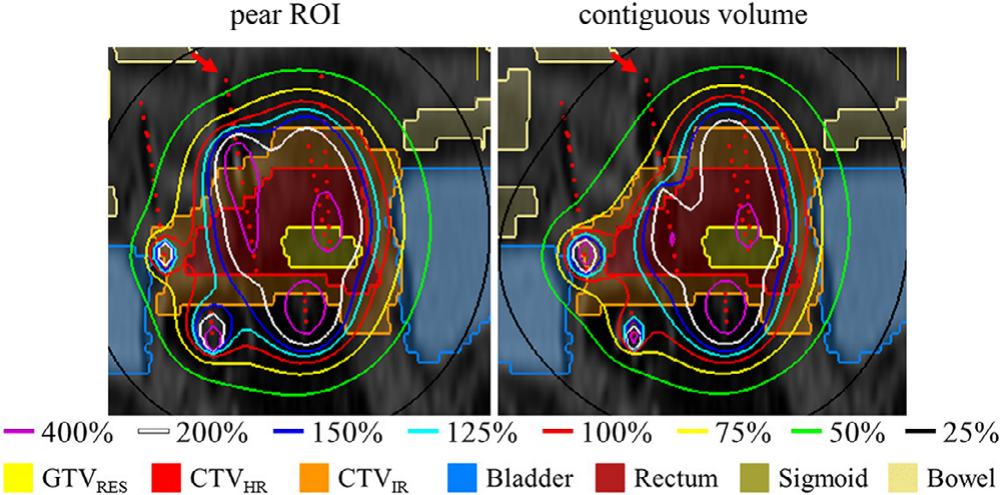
Dose distribution resulting from optimization with pear‐shaped ROI versus contiguous volume for a patient where a needle is used as the intrauterine part of the applicator in terms of dosage. The intrauterine applicator is marked by the red arrow. Coronal view along MRI axes, visualized with in‐house developed graphical user interface. MRI, magnetic resonance imaging; ROI region of interest.
